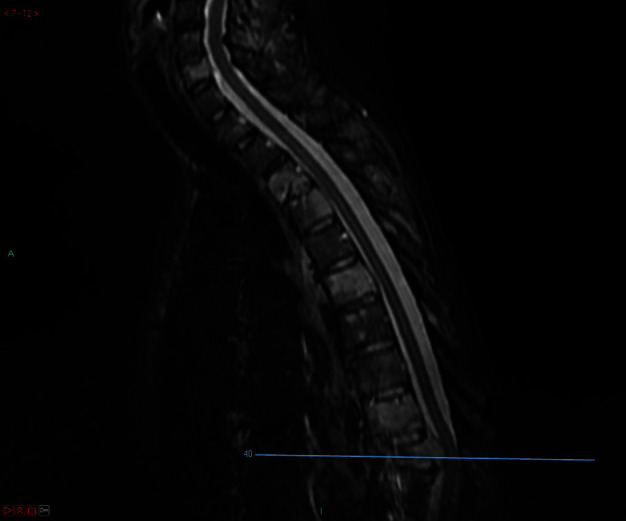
Preoperative MRI STIR (sagittal images, level of compression).
